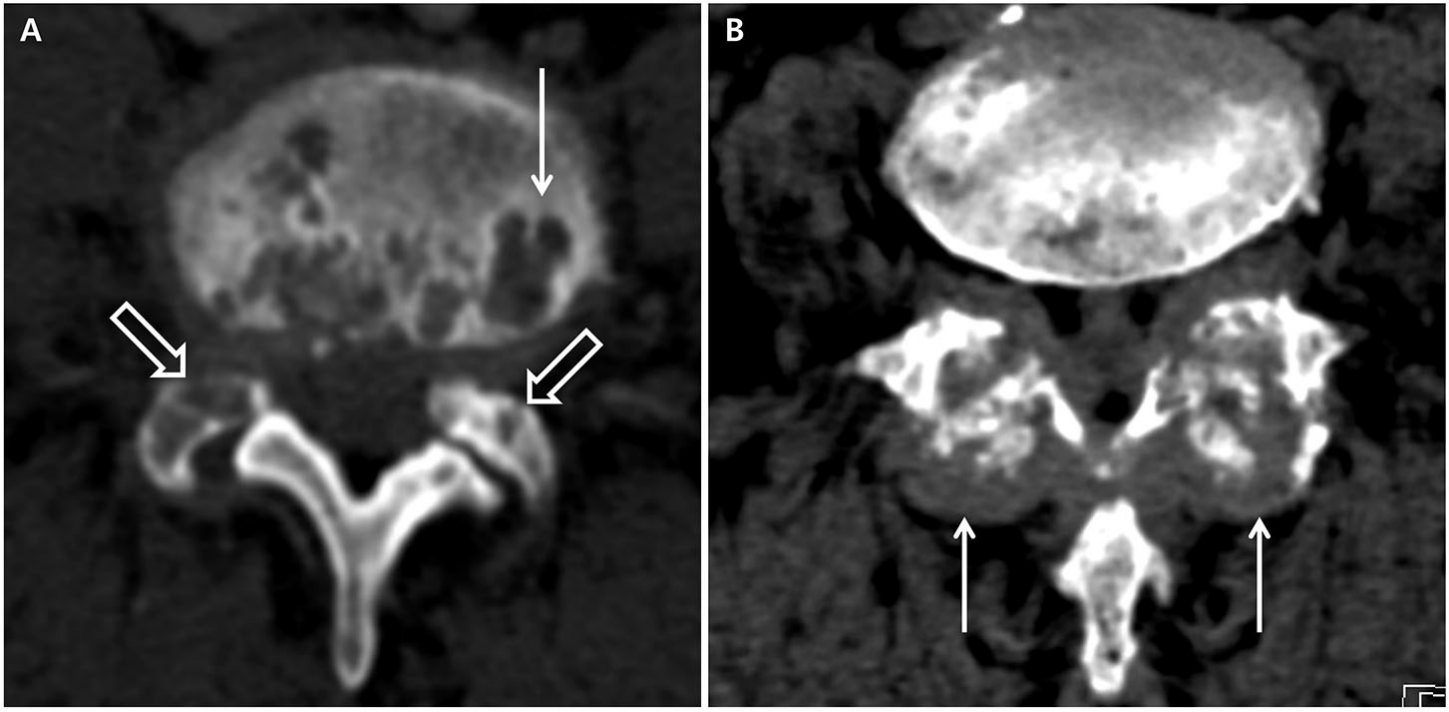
Computed tomography suggestive of axial gout. (A) Erosive changes with sclerotic margins in the vertebral body (white arrow) and facet joints (white hollow arrows). (B) Tophi in the facet joints (white arrows).
